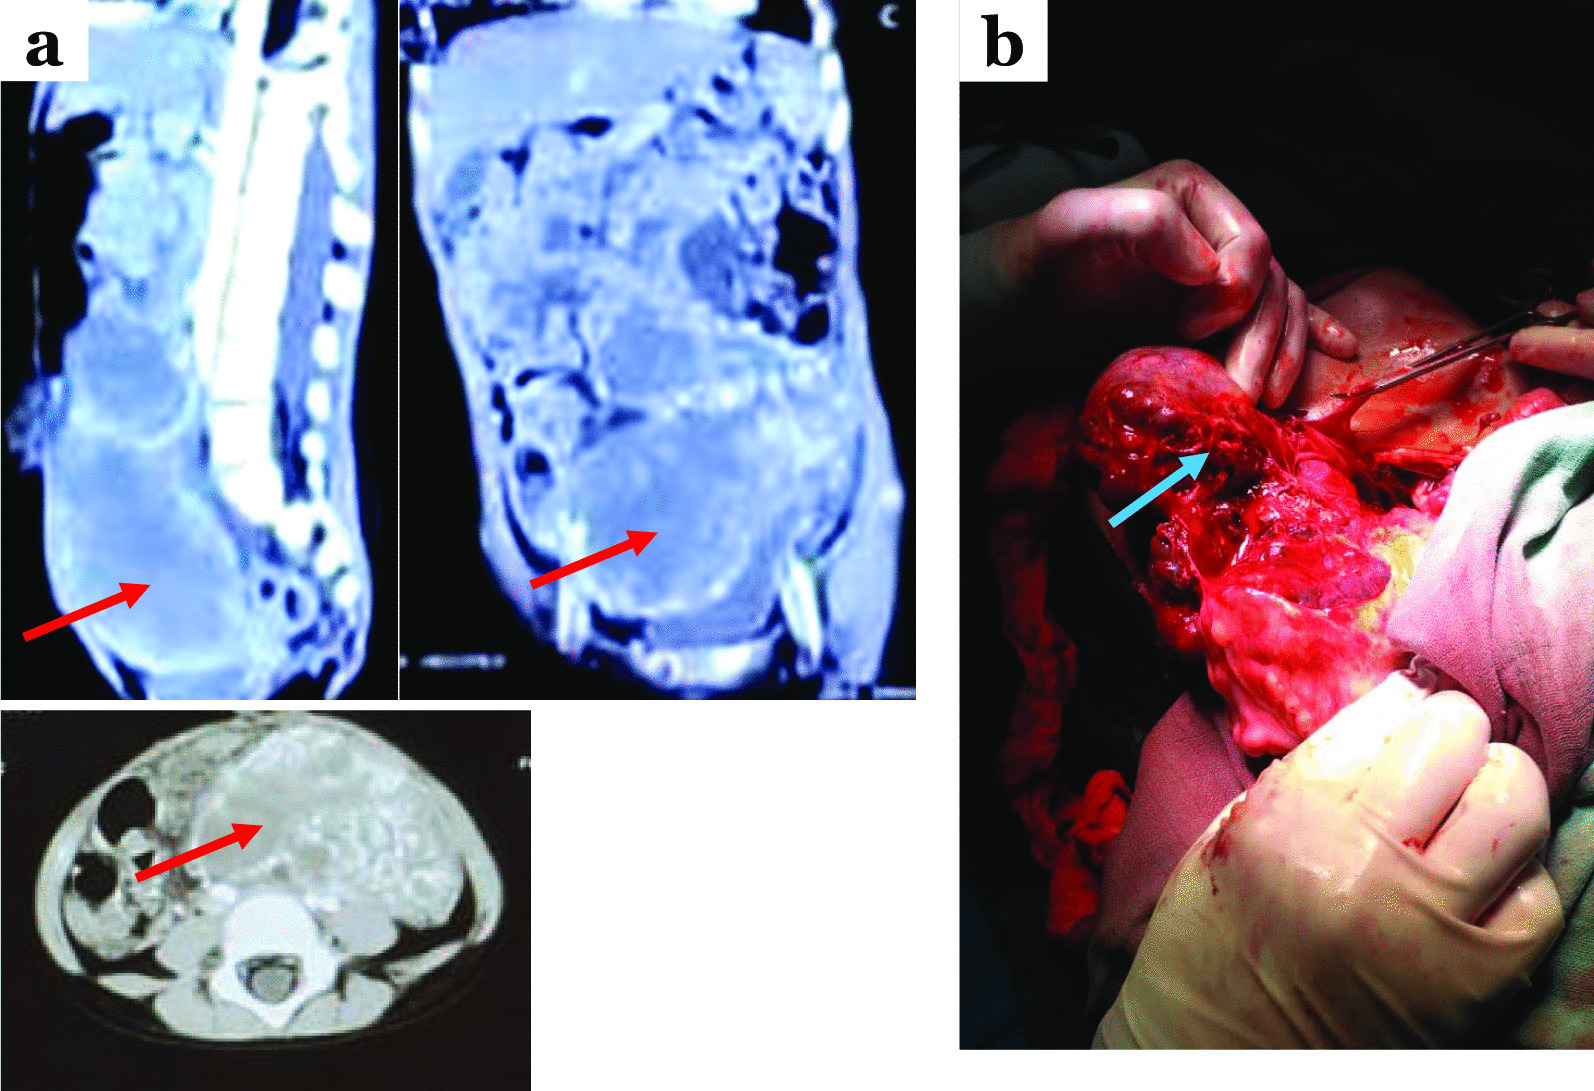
a Abdominal computed tomography scan showing a large mass (10 × 6 × 12 cm) with fluid density and calcification (red arrow), leading to the suspicion of left ovarian teratoma. b Intraoperative finding revealing a tumor originating from the uterus (blue arrow), not the ovarium
